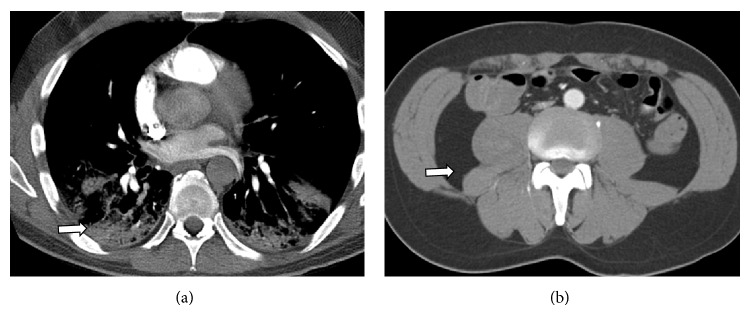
(a) CT chest on admission. Axial contrast enhanced images through the chest in the pulmonary phase demonstrate a normal main pulmonary artery trunk and normal segmental pulmonary arterial branches extending to the superior segment of the right upper lobe. Lung windows reveal lower lobe consolidation (see arrow). (b) Normal appearance of the retroperitoneum at that time (see arrow).
